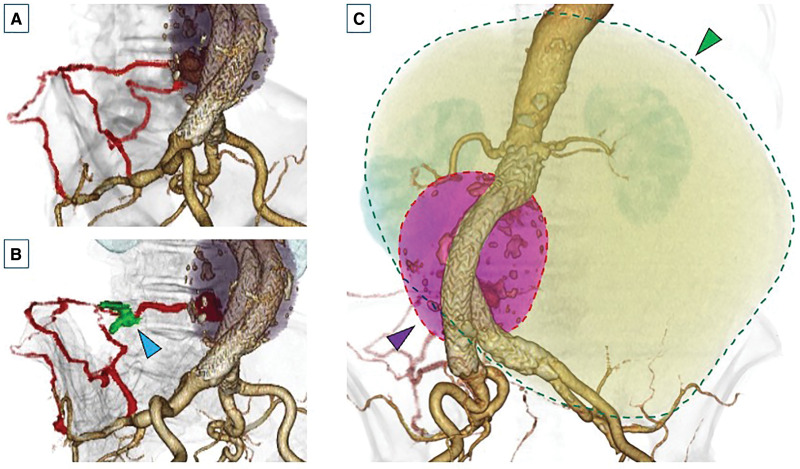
CT radiographs acquired one year ago. (A) Contrast-enhanced CT radiograph acquired one year ago before coil embolization, showing that the right L4 lumbar artery, which was the cause of the type II endoleaks, had developed collateral circulation from the right superior gluteal artery. (B) Contrast-enhanced CT radiograph acquired after coil embolization, showing that the coil advanced only halfway through the catheter and was embolized in the middle of the path (blue arrowhead). (C) Preoperative contrast-enhanced CT radiograph showing the diameter of the aneurysm sac to be 64 × 81 mm (red dashed line, purple arrowhead). The giant ovarian tumor had expanded, and its volume was 5381 cm^3^ (green dashed line, green arrowhead). The abdominal aortic aneurysm was displaced to the right side by the giant ovarian tumor.
